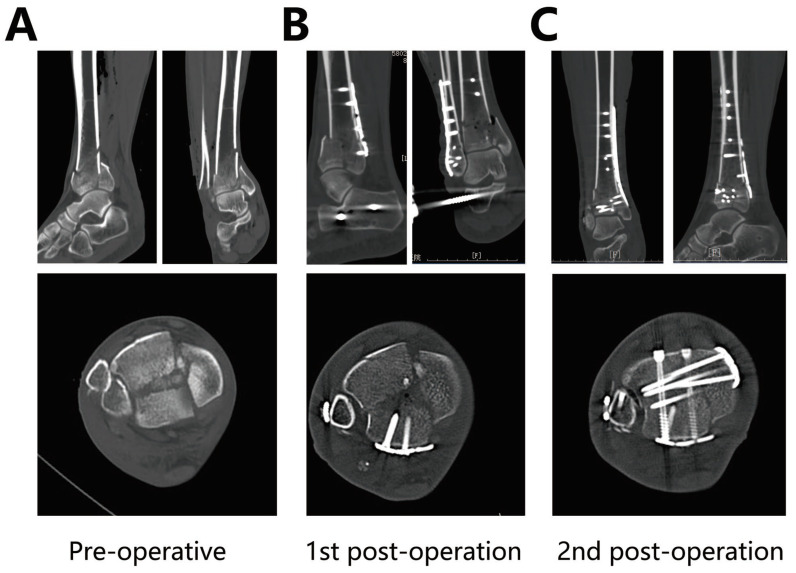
CT images of the patient (A) after the fracture (B) after the first surgery (C) after the second surgery.
